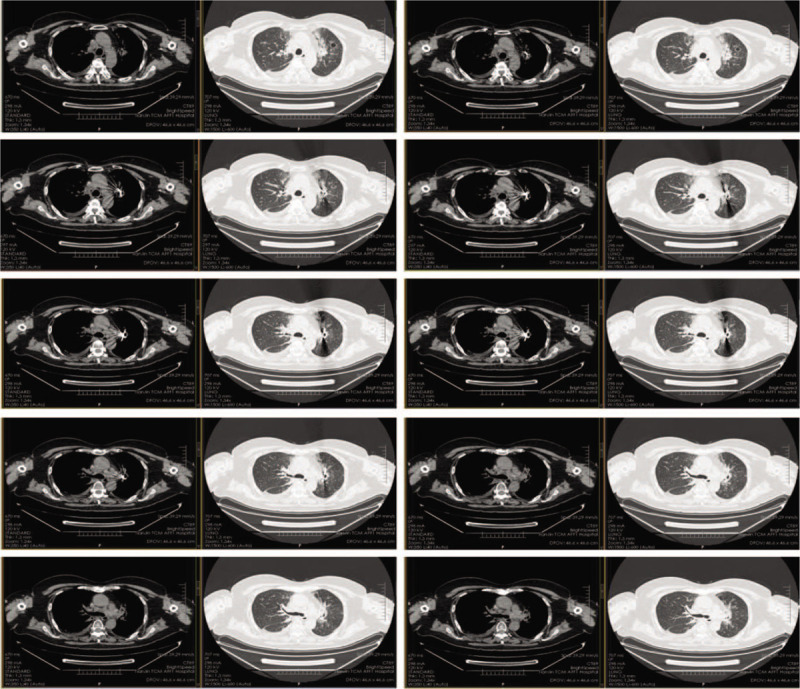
Chest computed tomography after iodine-125 radioactive seeds brachytherapy (June 22, 2018).
